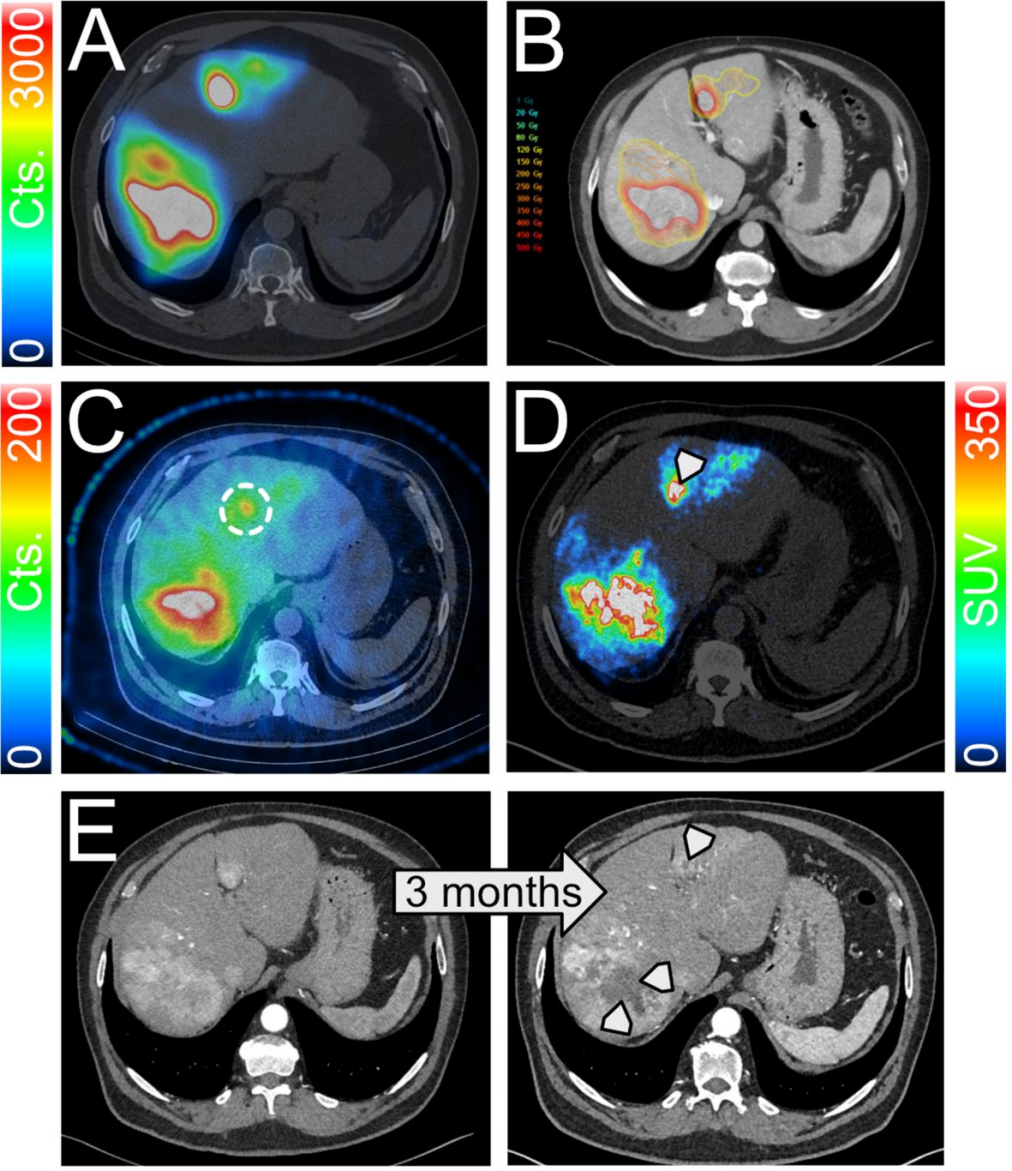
Pre-therapy MAA-distribution of 66-year-old patient with HCC in Segments 2 and 7 (A) together with the planned perfused tumor dose of 400 Gy (B). The post-therapy SPECT shows only minimal accumulation in segment 2, indicating under-treatment (C). The LAFOV PET images show clearly strong enhancement in all planned HCC manifestations (D). Confirming this, follow-up images after SIRT show central necrosis following the contours of the dose accumulations
